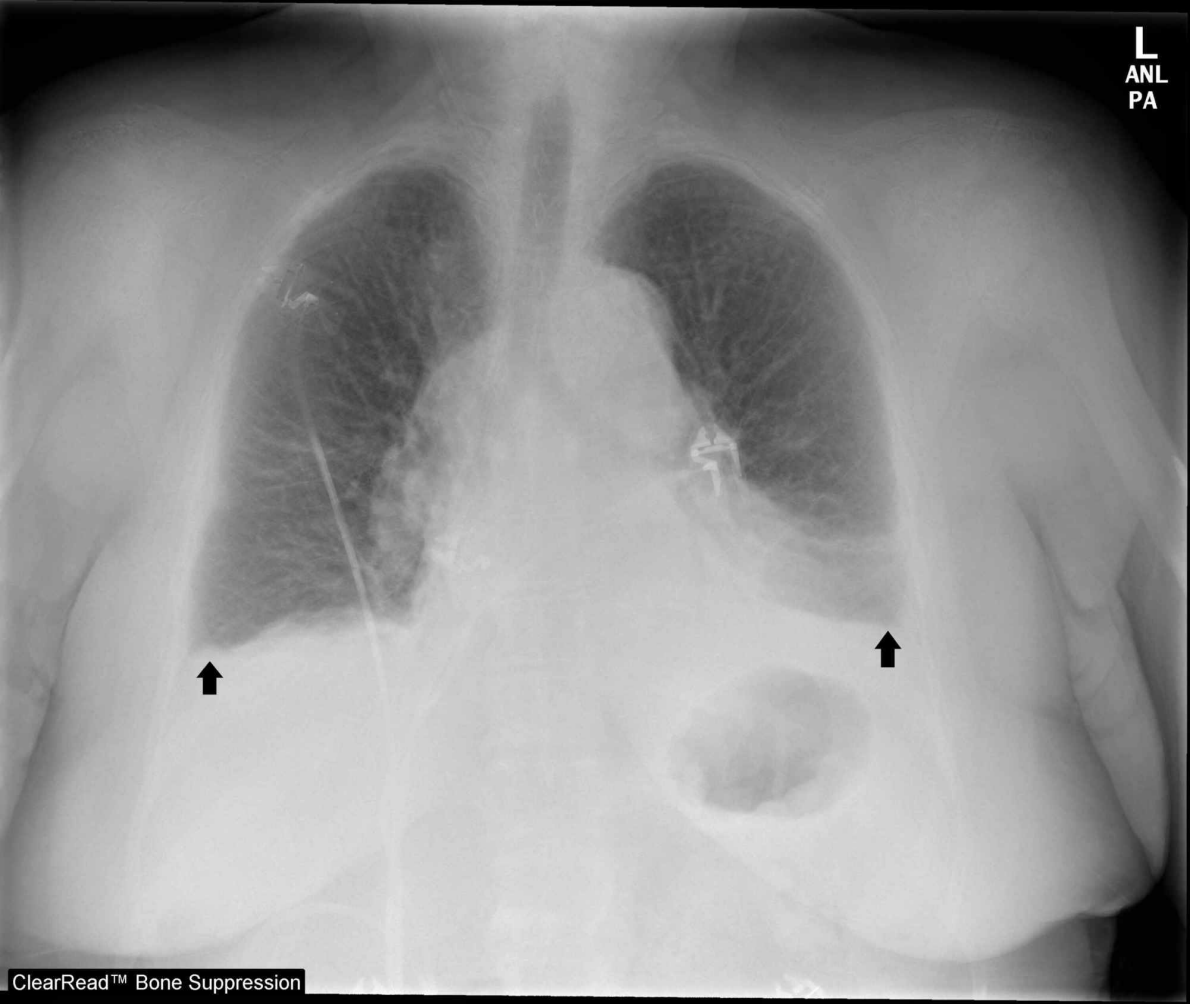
One Day Later, Repeat Chest Radiography (CXR) Black arrows identify bilateral pleural effusions.  CXR also notable for diffuse vascular congestion with pulmonary edema.
